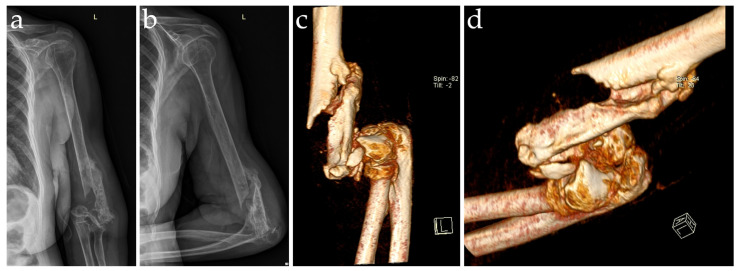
Preoperative anteroposterior (a) and lateral (b) radiographs and volume rendering technique CT images (c,d) of a 61-year-old female patient, showing left humeral pseudarthrosis. The patient was referred to our hospital due to a history of left distal humeral multiple traumas, resulting in left distal comminuted, bicolumnar, intra-articular fracture with displacement AO C2/C3 classification, Jupiter Y-type classification and left olecranon Mayo type II displaced non-comminuted stable fracture, AO type B intra-articular fracture, and Schatker simple transverse fracture type A. She underwent multiple open reduction and internal fixation surgeries with ulnar transposition, with medial and lateral plates with screws for the distal humerus fracture and a tension band technique with K-wires for the olecranon fracture, at another hospital. Subsequently, she experienced another traumatic event on the same arm after falling from a height. As a consequence, a peri-implant fracture occurred, alongside the failure of the internal fixation device, leading to the removal of the osteosynthesis materials. The patient exhibited left arm pseudarthrosis, impairing normal function of the elbow joint with persistent pain at the site of the fracture. The patient was referred to our hospital one year after the last surgical procedure. Clinical tests did not highlight any ulnar nerve injury, with no sensory dorsal or palmar dysfunction, no ulnar claw deformity of the hand, and normal motor function. The images show normal proximal radio-ulnar morphology. A CT examination with three-dimensional reconstructions was performed in order to assess the damage to the left distal bone stock and the state of deep tissue fibrosis, as well as to approximately calculate the length of the missing bone segment and the size of the distal humeral reconstruction segment. Both CT exams and X-rays showed severe elbow arthritis, with significant joint space narrowing, osteophytes found at the olecranon tip, and loose bodies. After thorough consideration and analysis in a multidisciplinary board and ruling out subclinical infections by using biological markers and tissue biopsy with joint aspiration, the recommended therapeutic solution was a megaprosthesis, which is usually reserved for tumoral cases but was considered the best approach for this patient [1,2]. The literature on the use of megaprostheses for non-neoplastic indications is scarce, and this novel approach has only been previously described in a handful of very recent cases [3,4]. Poor bone quality and multiple surgeries without clinical neurological deficiency were the main indications for the selected implant. Osteosynthesis, bone reduction, bone grafting, and fixation were not considered as solutions because of previous failures and the possibility of large avascular distal fragments of the humeral shaft that made bone integration unlikely.
